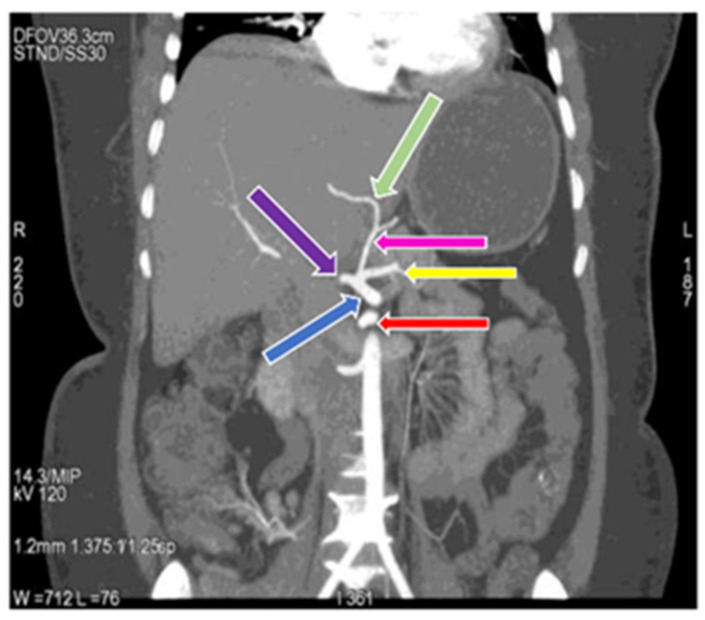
Coronal MIP image of (64 row scanner CT angiography) abdominal region showing the celiac trunk (blue arrow) and superior mesenteric artery (red arrow), the celiac trunk gives rise the left gastric artery (pink arrow), common hepatic artery (purple arrow) and splenic artery (yellow arrow), in which left gastric artery gives rise to left hepatic artery (light green arrow). Slice thickness: 1.2 mm.
